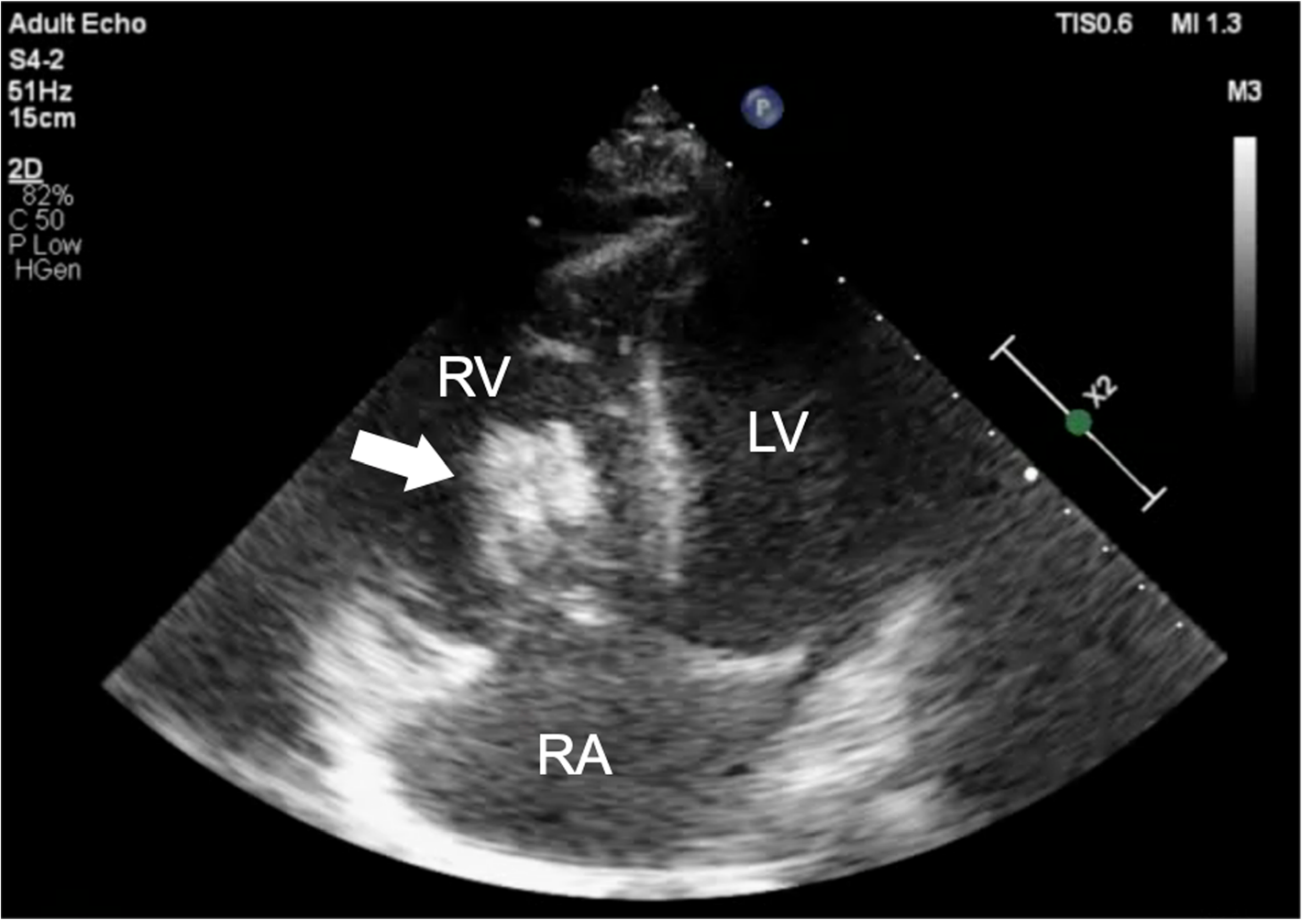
Transthoracic echocardiography on readmission showing right ventricular dilatation and a mobile mass attached to the tricuspid valve (arrow). RV: right ventricle, LV: left ventricle, RA: right atrium
